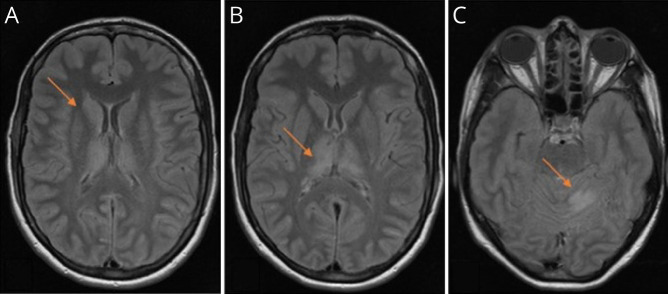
Brain MRI From the Index Patient MRI performed 12 days after symptoms onset, during an ongoing myoclonic status. Fluid-attenuated inversion recovery T2 sequences showing hyperintensities in the right N. caudatus (A) as well as in both thalami (B) and in the cerebellum (C), compatible with an ongoing status epilepticus.
